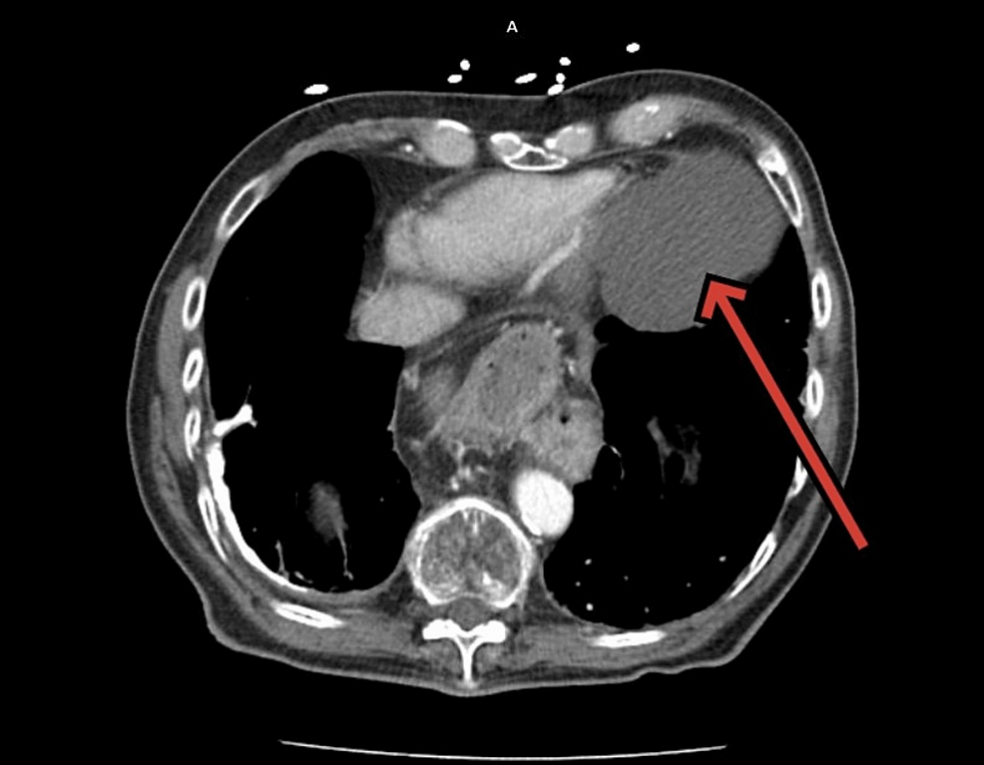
CT scan in transverse section showing pericardial cyst (red arrow)
